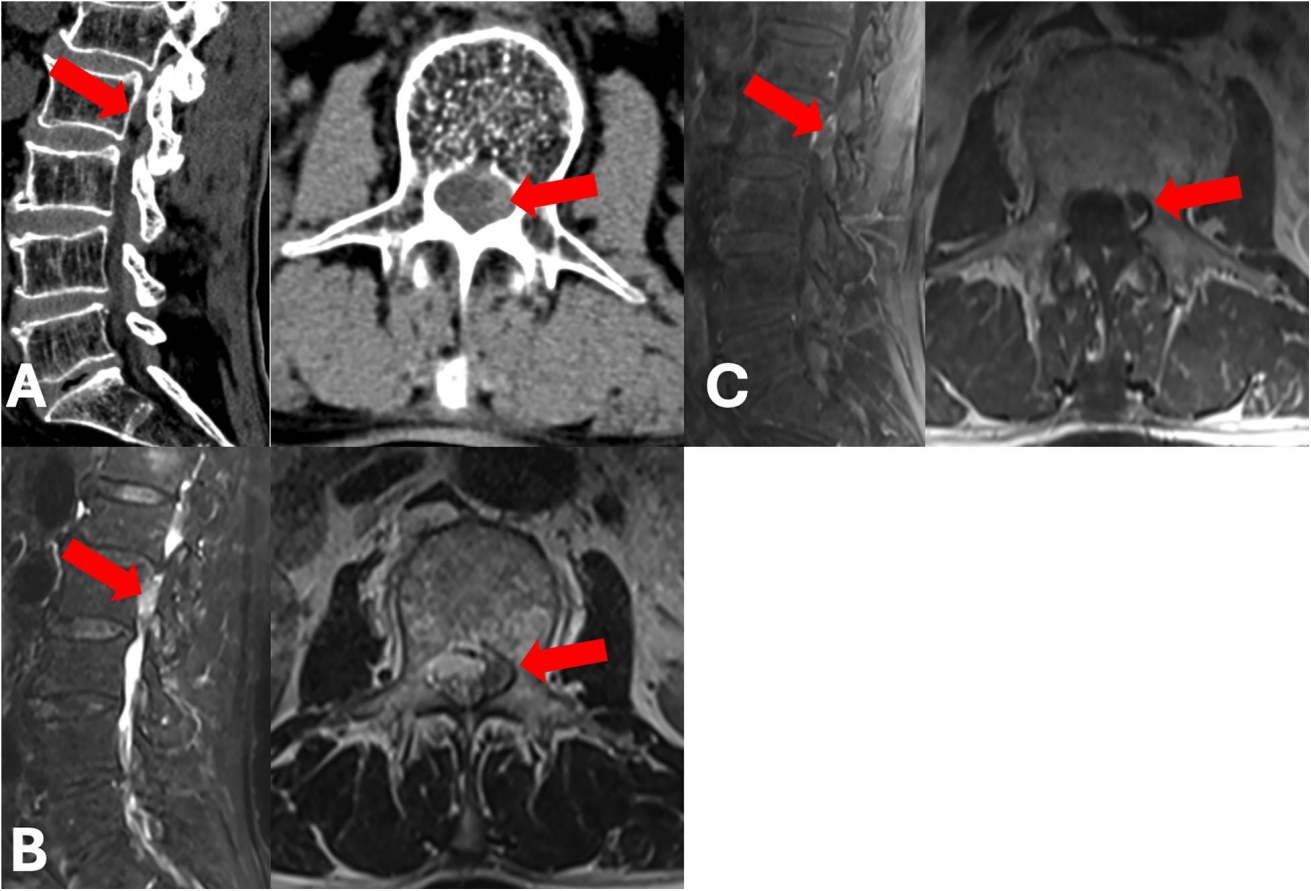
Preoperative imaging studies. The herniated disc is indicated by red arrows. (A) Preoperative computed tomography (CT) scan. (B) Preoperative magnetic resonance imaging (MRI) scan. (C) Preoperative contrast-enhanced MRI scan.
